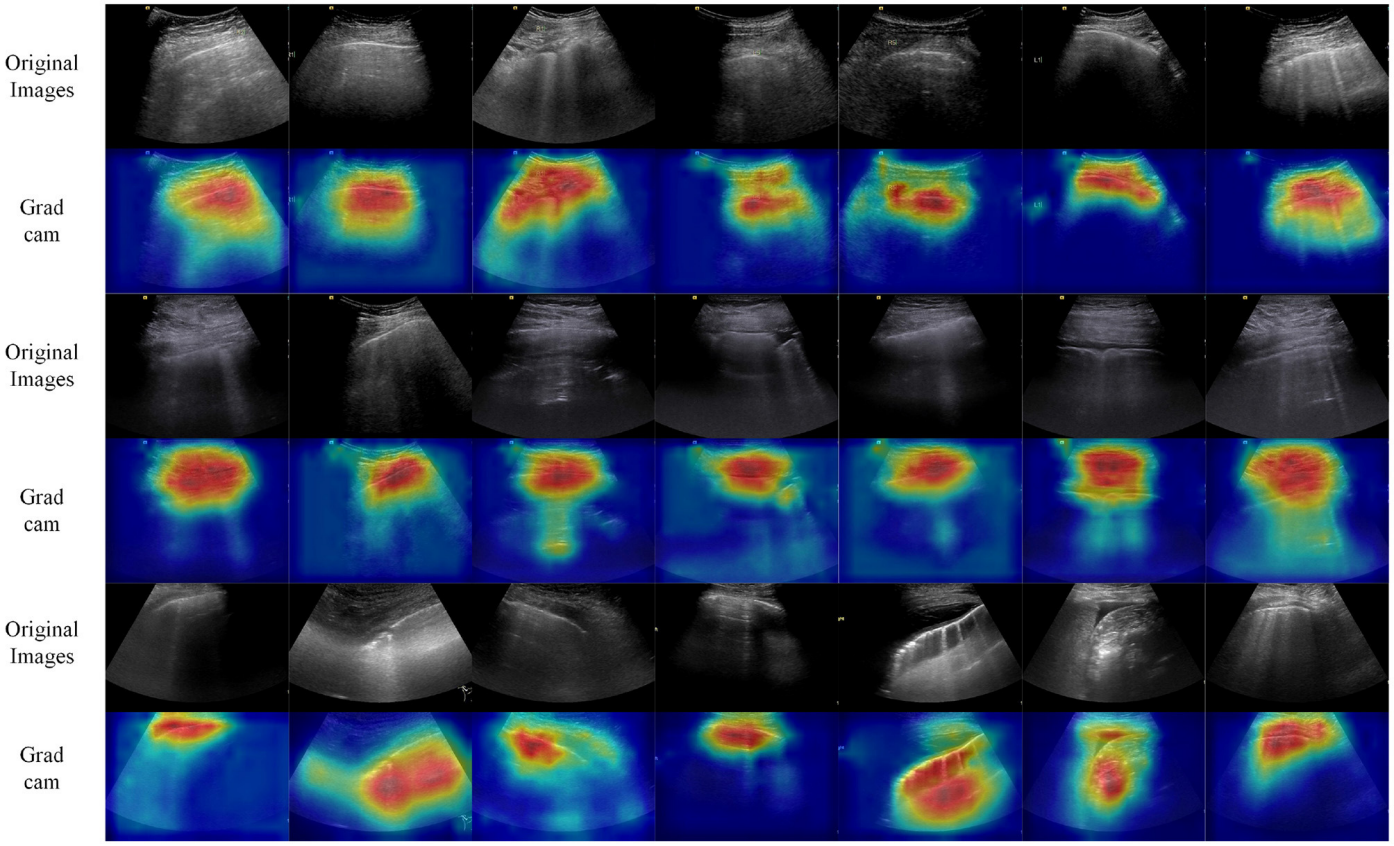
Nidus-related visualization of ultrasound images. Using grad-CAM (44), the proposed model could highlight the image areas that are most relevant to COVID-19.
